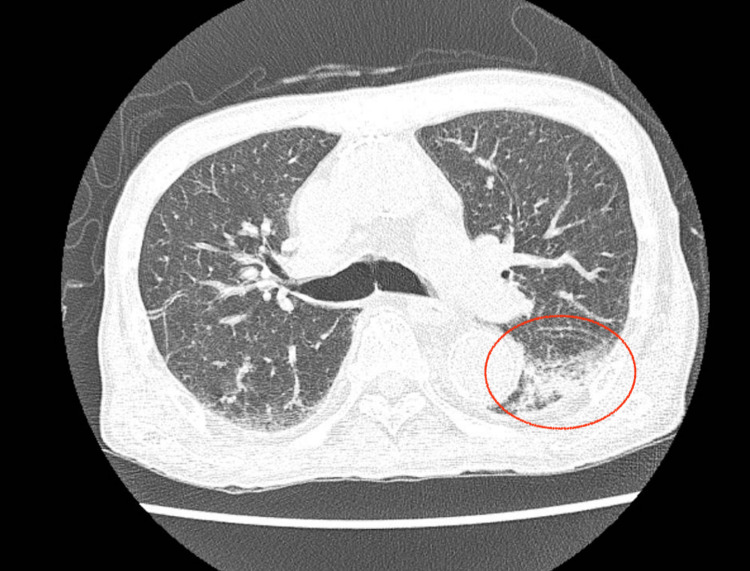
CT findings showing ground-glass opacity in the left lung (red circle)
